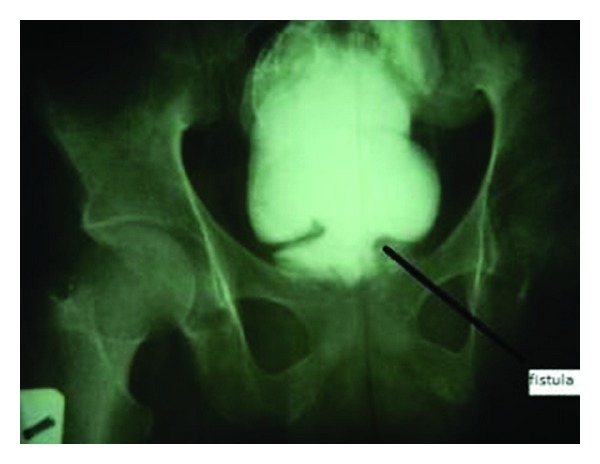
Micturating cystourethrogram radiograph showing contrast intravasation into the sigmoid colon during the filling phase of the cystography. Black arrow pointing at the vesicocolonic junction.
